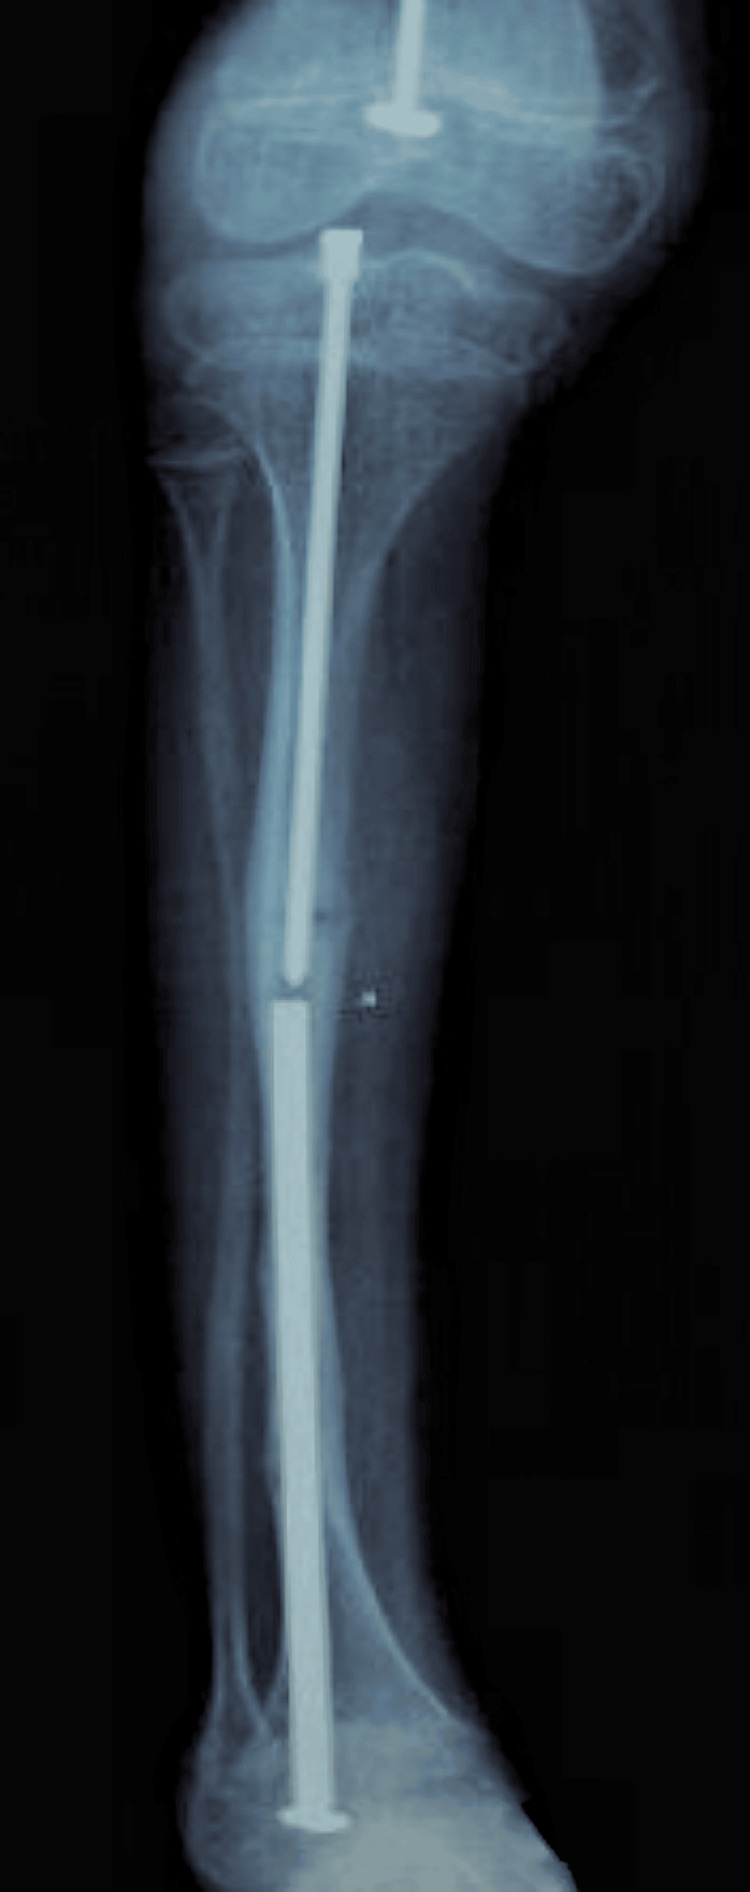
Radiograph showing complete premature opening of the telescopic nail This outcome may compromise stability and require revision surgery.
